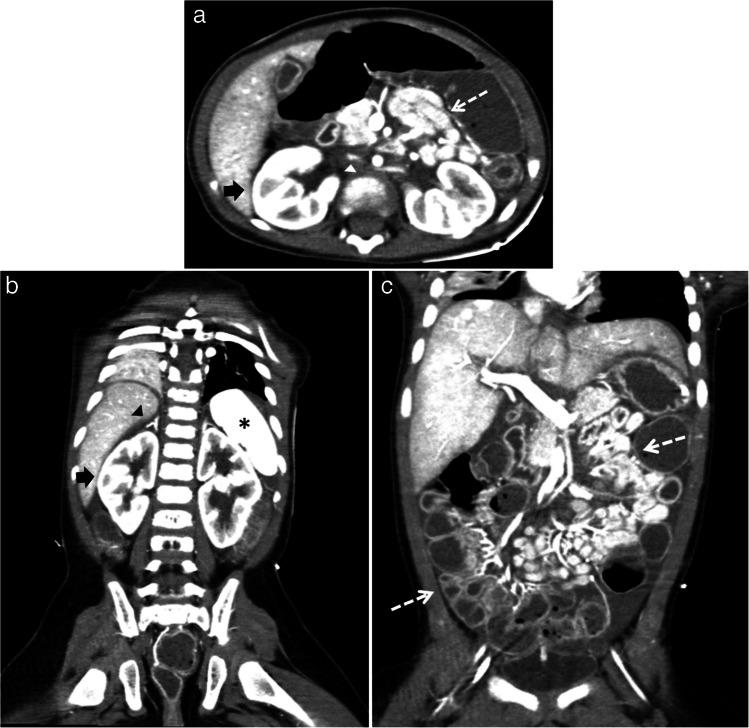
An 11-month-old female found unresponsive after reportedly falling off a couch at home. CT head found significant traumatic brain injury. Further trauma work was obtained with CT of the abdomen and pelvis with IV contrast in the portal venous phase, which demonstrated a constellation of findings consistent with hypoperfusion complex. a Axial image shows hyperdense kidneys (*black arrow*), hyperdense bowel (*dashed white arrow*), and intensely enhancing, small caliber IVC (*white arrowhead*). b Coronal image shows hyperdense kidneys (*black arrow*), adrenal glands (*black arrowhead*), and spleen (*asterisk*). c Coronal image of the same study at a different plane shows fluid-filled and hyperdense bowel wall (*dashed arrow*). Note the intensely enhancing mesenteric vessels, which extend into the serosal margin of bowel loops. In summary, trauma workup discovered multiple injuries including subdural hemorrhages, bilateral retinal hemorrhages, healing rib fractures, liver laceration, and bruising to the face and body, consistent with an inflicted abusive injury mechanism. The patient was declared brain dead and expired within 48 hours of presentation
